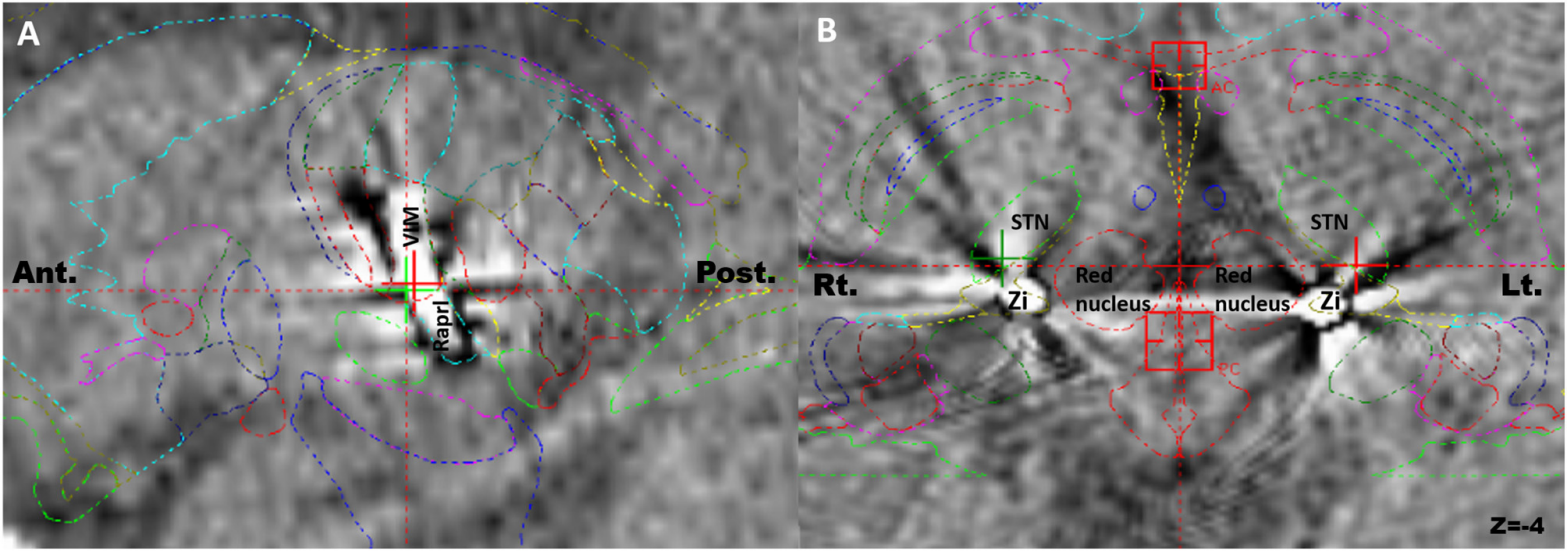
Postoperative computed tomography scans merged with preoperative magnetic resonance images for identification of the actual electrodes and active contacts using Schaltenbrand atlas. (A) The electrode hit the ventralis intermedius and the prelemniscal radiation simultaneously in the sagittal plane. (B) The tips of bilateral electrodes were located in the zona incerta in the axial plane. Ant, anterior; Post, posterior; Rt, right; Lt, left.
